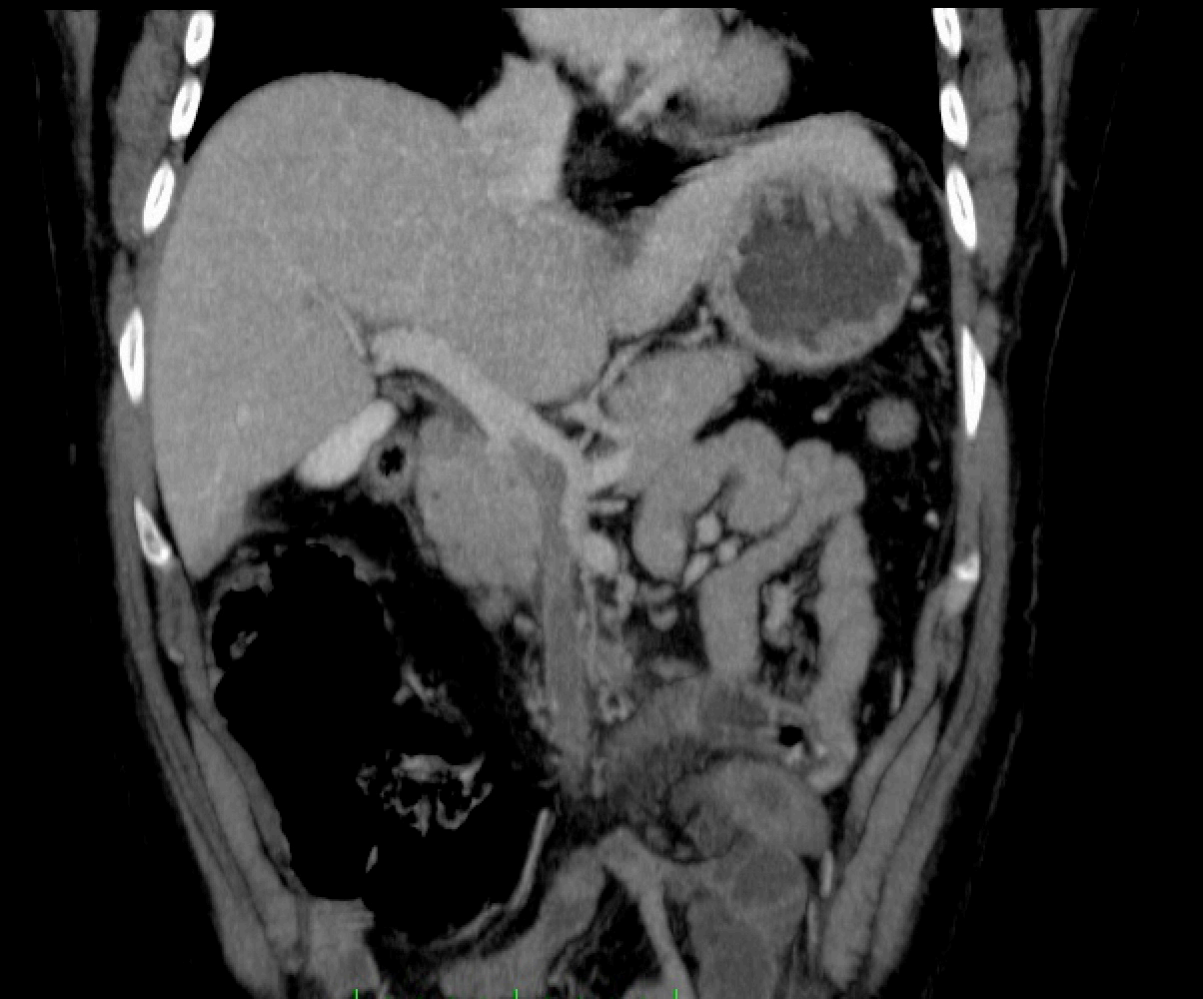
Computed tomography angiography of the abdomen and pelvis, showing images suggestive of failure to fill the interior of portal and superior mesenteric veins, compatible with presence of thrombi..
